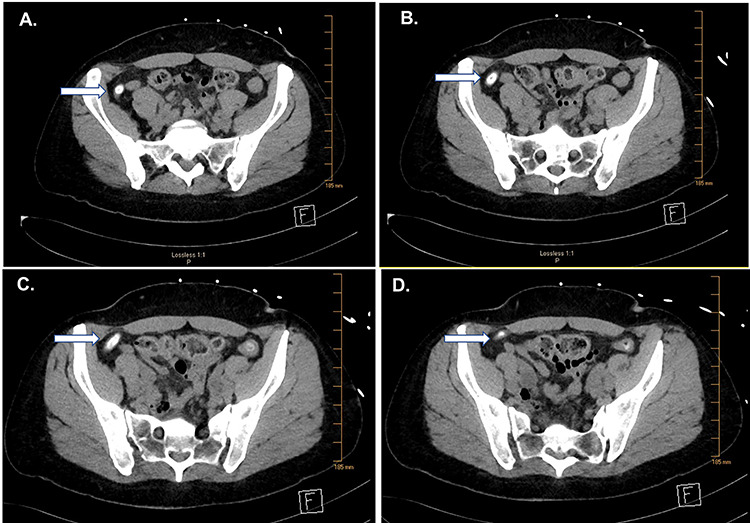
CT of the abdomen and pelvis. Serial images (A–D) from cephalad to caudal show a large fecalith (arrows).
